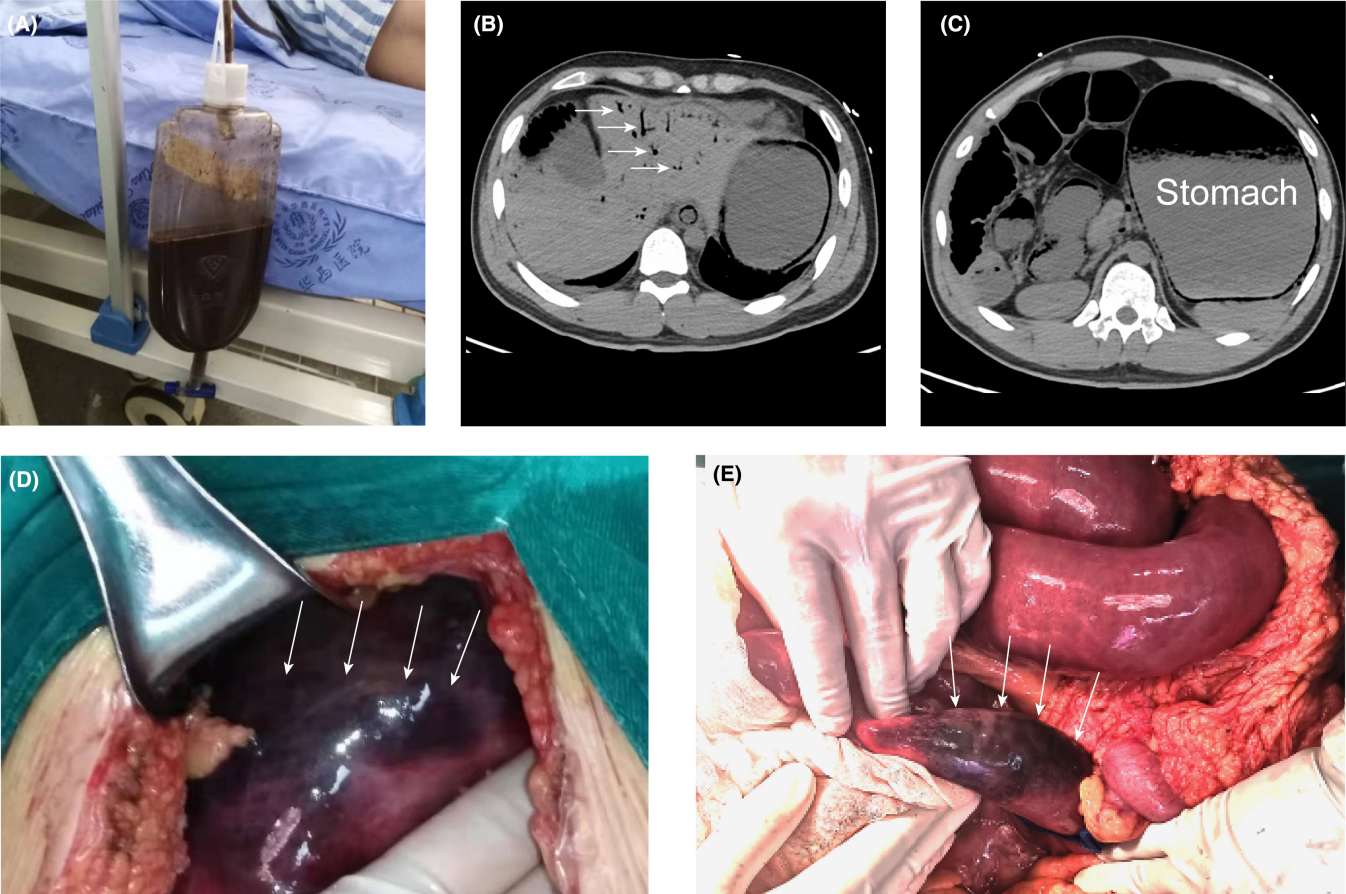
(A) Dark‐red bloody stomach content in the gastrointestinal decompression apparatus; computed tomography of the abdomen demonstrating (B) hepatic portal venous gas sign (arrow) and (C) severe gastrointestinal tract dilatation. (D, E) Intraoperative photographs revealing gastrointestinal tract necrosis from cardia to proximal 15 cm of the jejunum (arrow).
